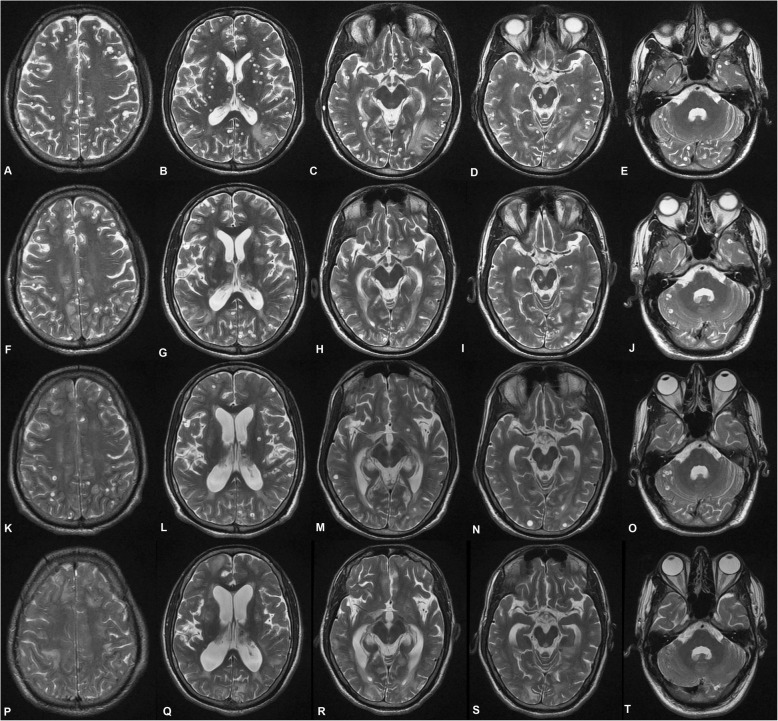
Axial T2W MRI images from another patient of disseminated cysticercosis. a-e Baseline images at different levels. f-j First follow-up at the same levels. k-o Second follow-up at the same levels. p-t Third follow-up at the same levels. A graded reduction can be noted from baseline to third follow-up. a, f, k and p Centrum semiovale level. b, g, l, and q Basal ganglia level. c, h, m, and r First mesencephalic cut. d, i, n, and s Second mesencephalic cut. e, j, o, and t Middle cerebellar peduncle level
